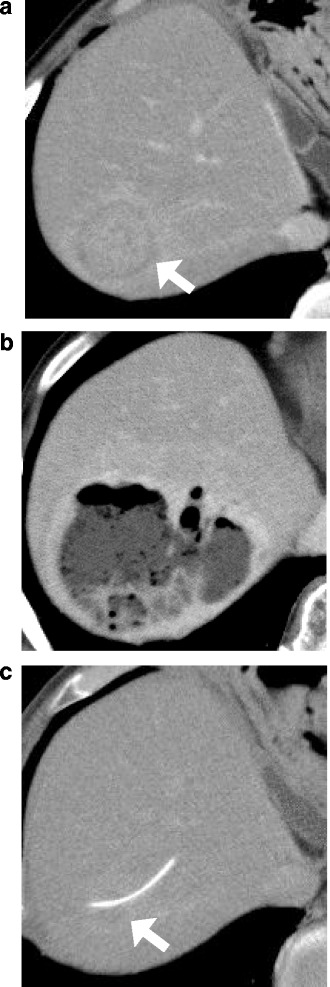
Enhanced computed tomography (CT) at initial diagnosis (a), two months after steroid initiation (b), and after percutaneous drainage placement (c). Initial CT showing a 5 cm-sized hepatic mass in segment 7 (a). Following CT after steroid initiation showing a 9.5 cm, multilocular abscess replacing the inflammatory pseudotumor and containing fluid and gas with marginal enhancement (b). CT after percutaneous drainage placement showing a minimized abscess lesion (c)
